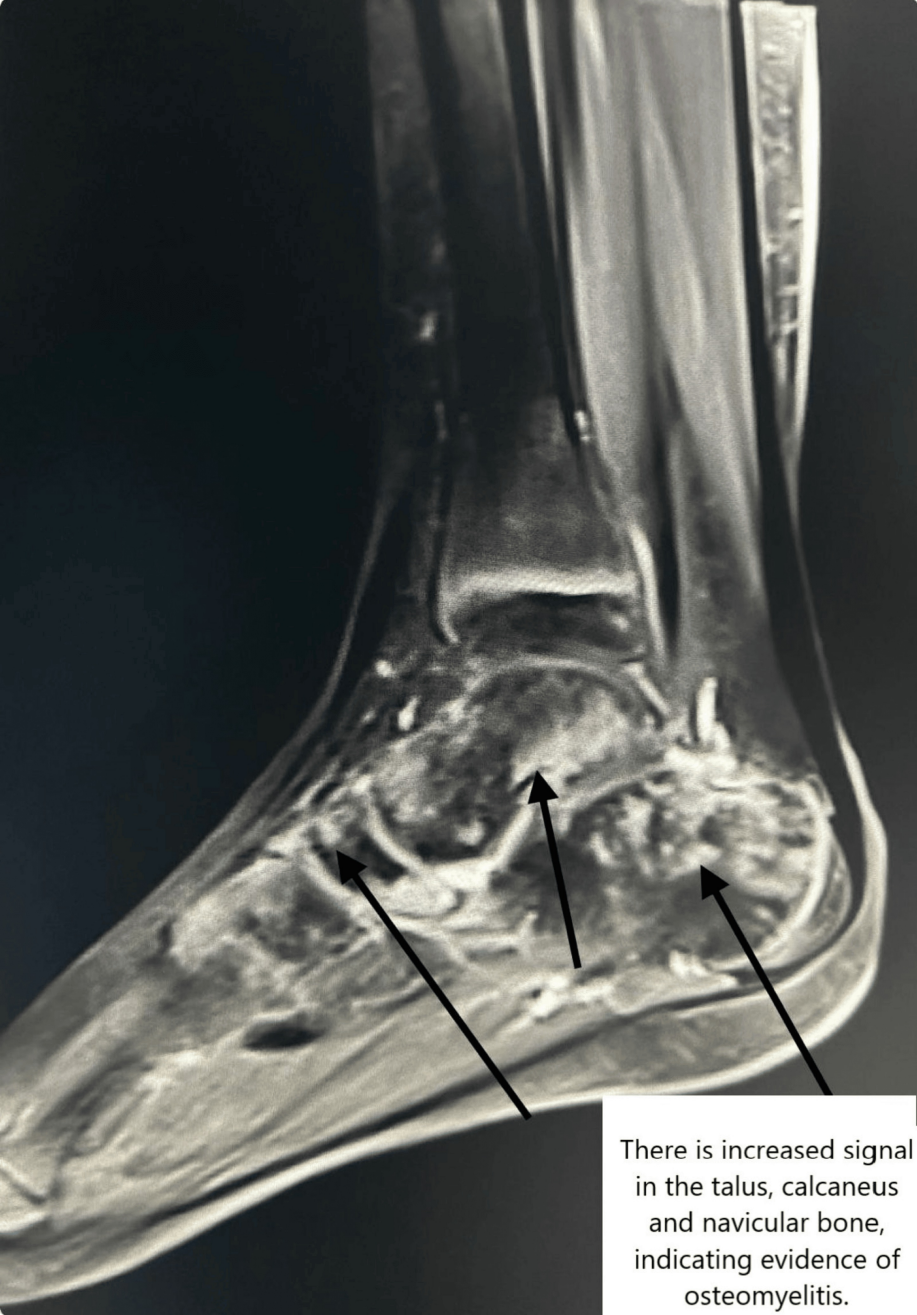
Magnetic resonance imaging revealed increased signal in the talus, calcaneus, and navicular bones of the right foot, suggesting osteomyelitis (arrows).
